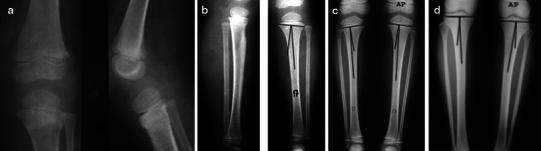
a Anteroposterior and lateral radiographs of a 5 year old boy with a Salter–Harris type II proximal physeal injury of the left tibia. b One year post-injury, with a valgus deformity of 4° compared to the normal side. c Three years post-injury; almost complete remodeling is observed. d Twenty-two years post-injury, without any angular deformity
